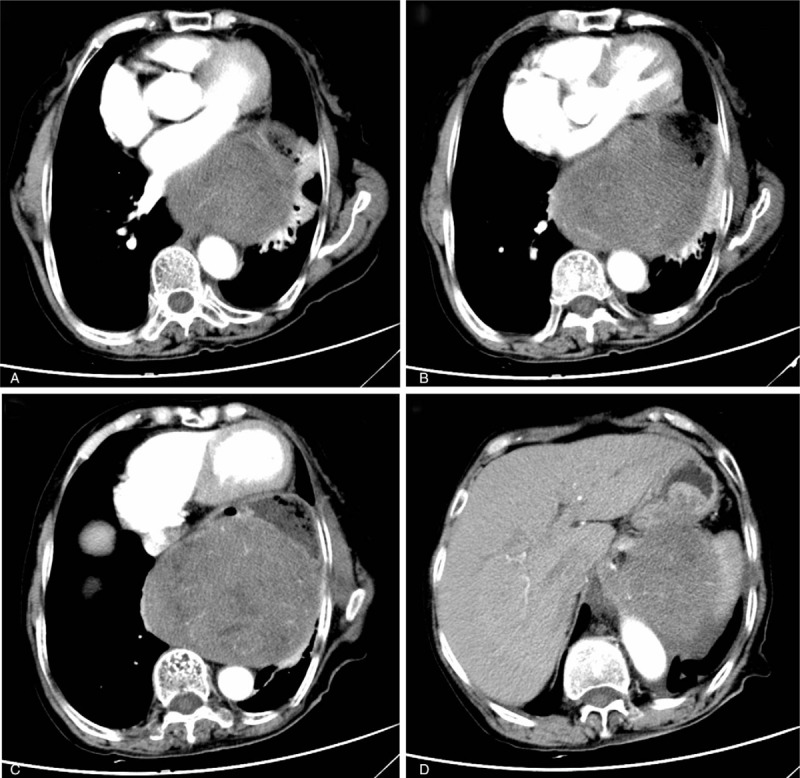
Contrast-enhanced abdominal computer tomography (CT) showed a heterogeneous, 11.9 × 10.2 cm posterior mediastinal tumor in the left lower thorax, thrusting the distal esophagus, pylorus, cardiac forward, pressing the pulmonary leading to the atelectasis of part of the upper and inferior lobe of left lung, and adhering to the lower esophagus, pylorus, and the left adrenal gland with unclear boundaries.
